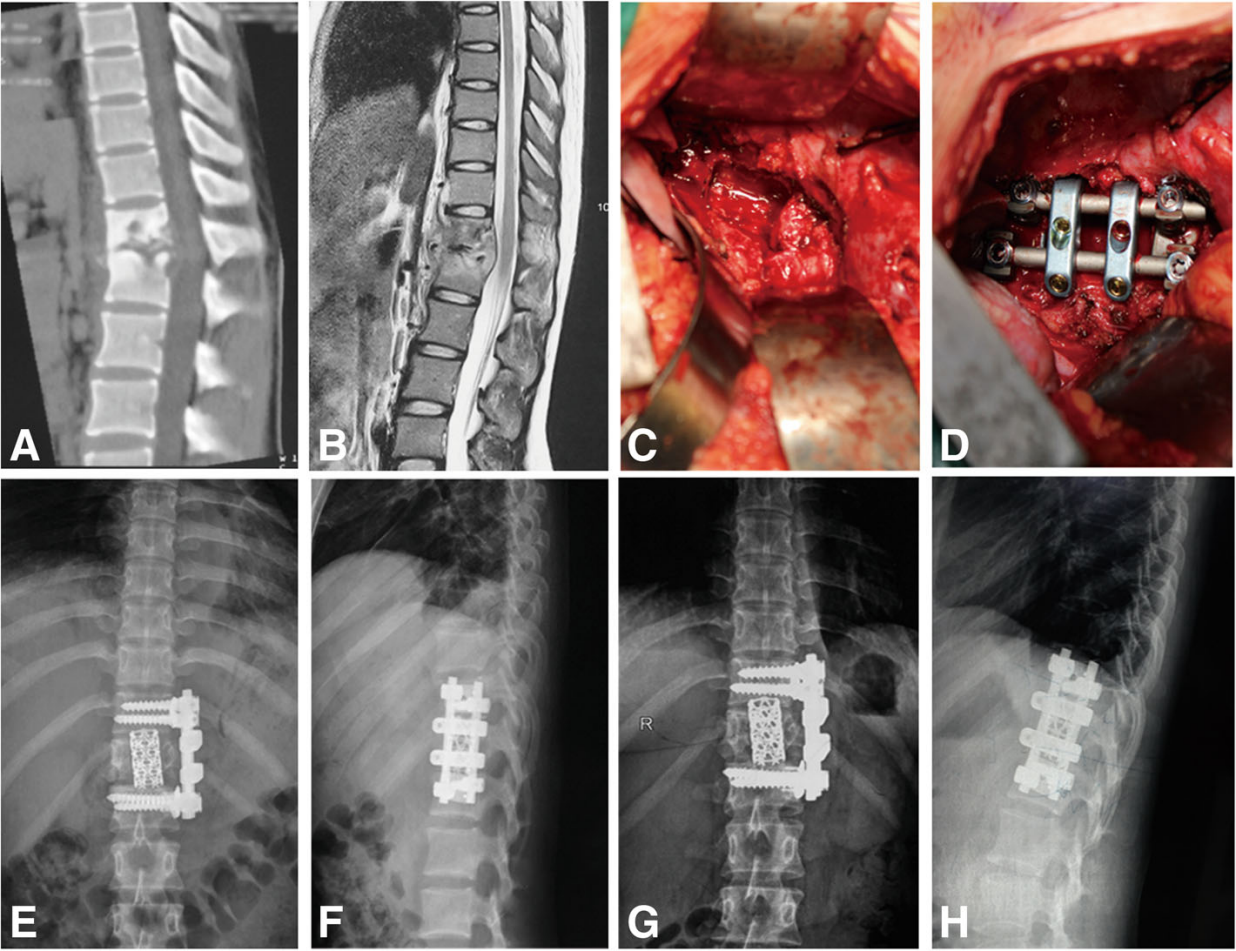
Twenty-year-old girl with tuberculosis (T12-L1 level). a Preoperative computed tomography scans demonstrate vertebral body destruction. b Preoperative magnetic resonance imaging scan shows vertebral body destruction and abscess. c, d Intraoperative debridement, titanium cage strut fusion, and internal fixation. e, f Postoperative radiographs show well-positioned internal fixation. g, h Radiographs showing satisfactory focal clearance and strut graft stability at the final follow-up
